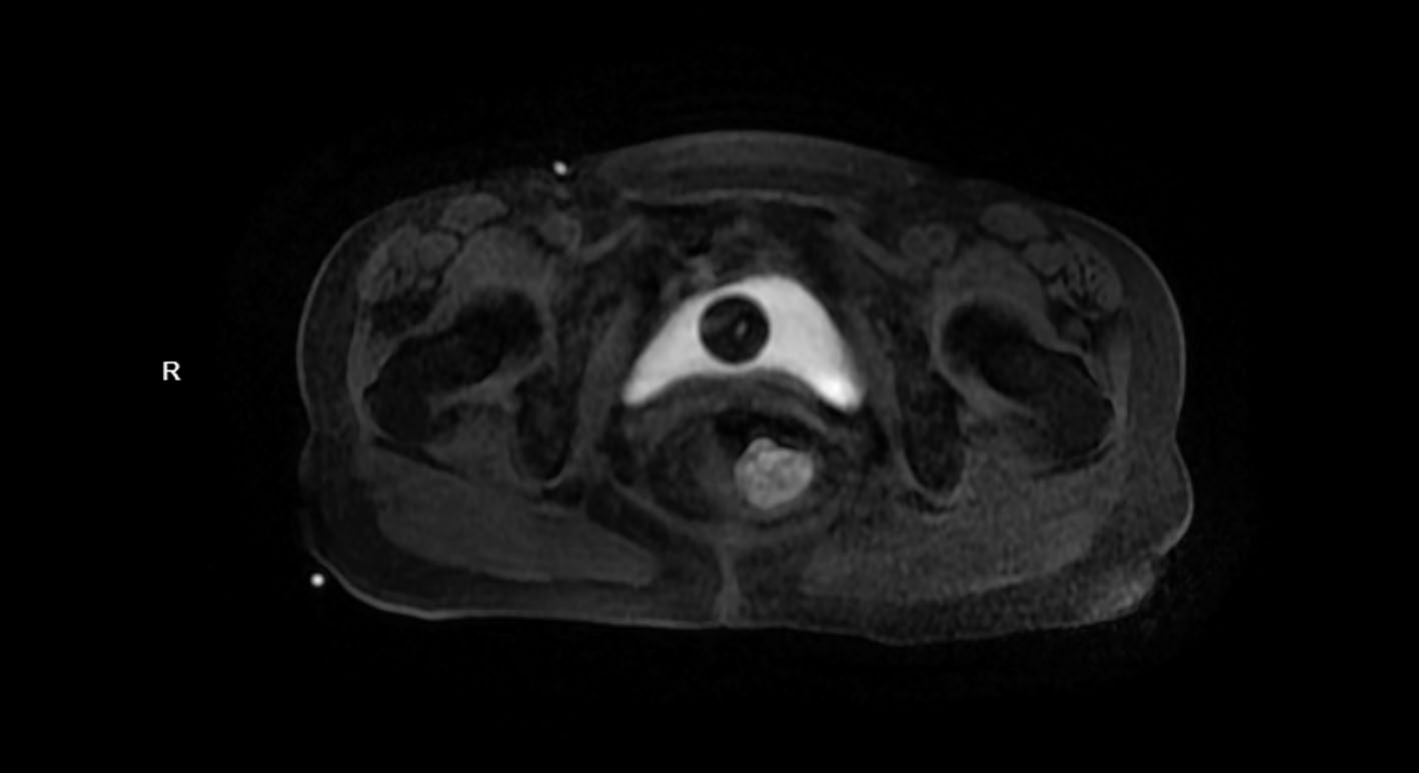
Imaging studies: Pelvic magnetic resonance imaging (MRI) with contrast enhancement revealed marked thickening of the right rectal wall, with a protuberant nodular lesion measuring approximately 32 × 27 mm
